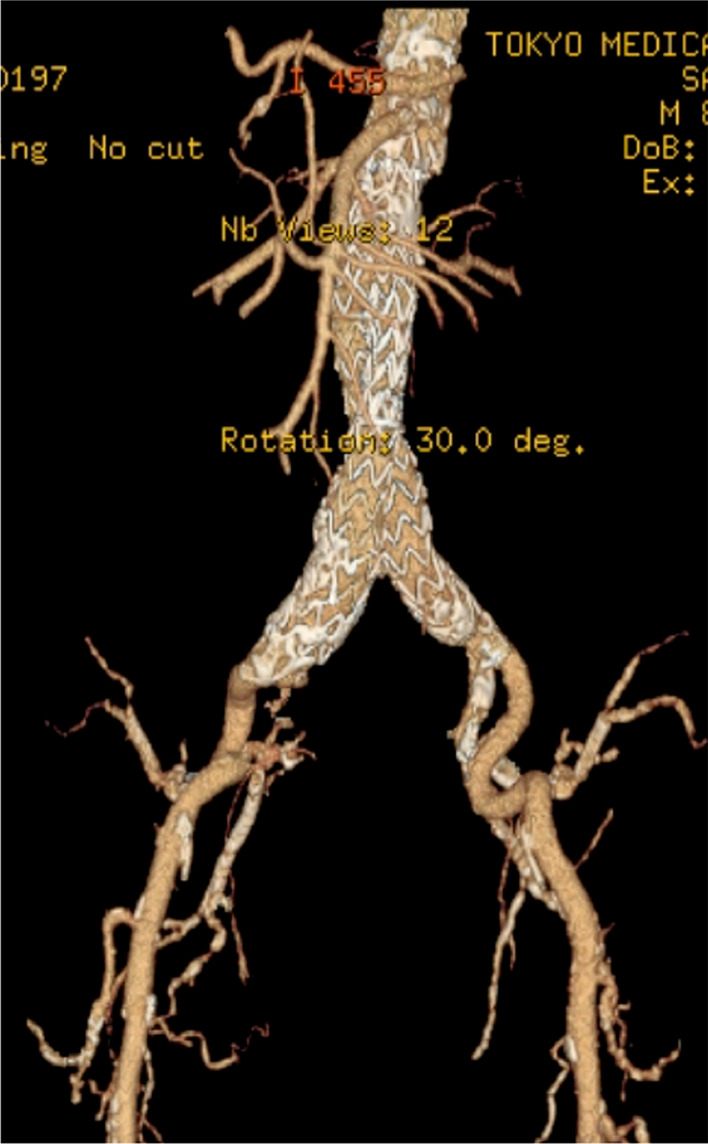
Contrast-enhanced CT showing an oozing of contrast medium on the main body and left limb
